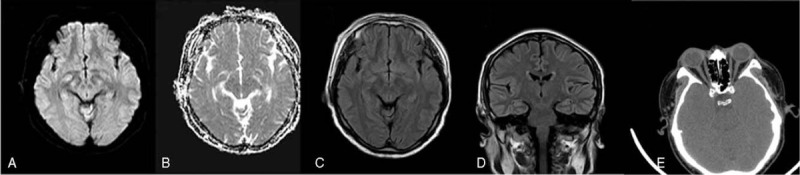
A–D. No obvious abnormality was found in emergency MRI; E. No obvious abnormality in orbital CT. CT = computed tomography, MRI = magnetic resonance imaging.
